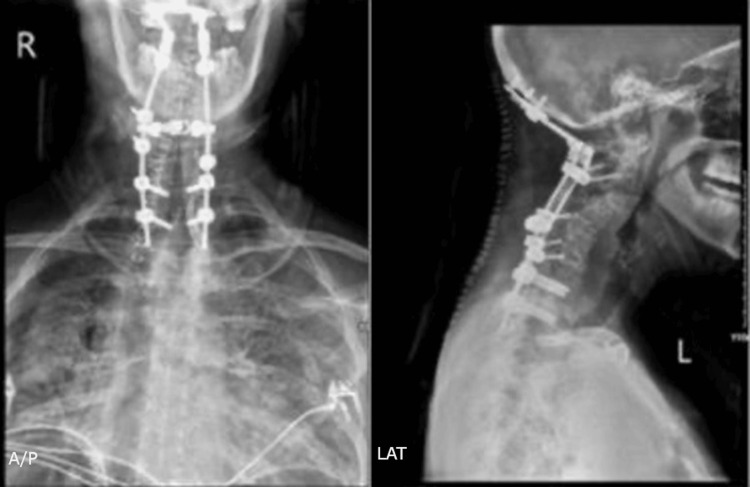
Postoperative Upright Cervical Radiographs Postoperative upright cervical radiographs demonstrating posterior occipitocervicothoracic instrumented fusion with partial deformity correction. A/P: anteroposterior; LAT: lateral
